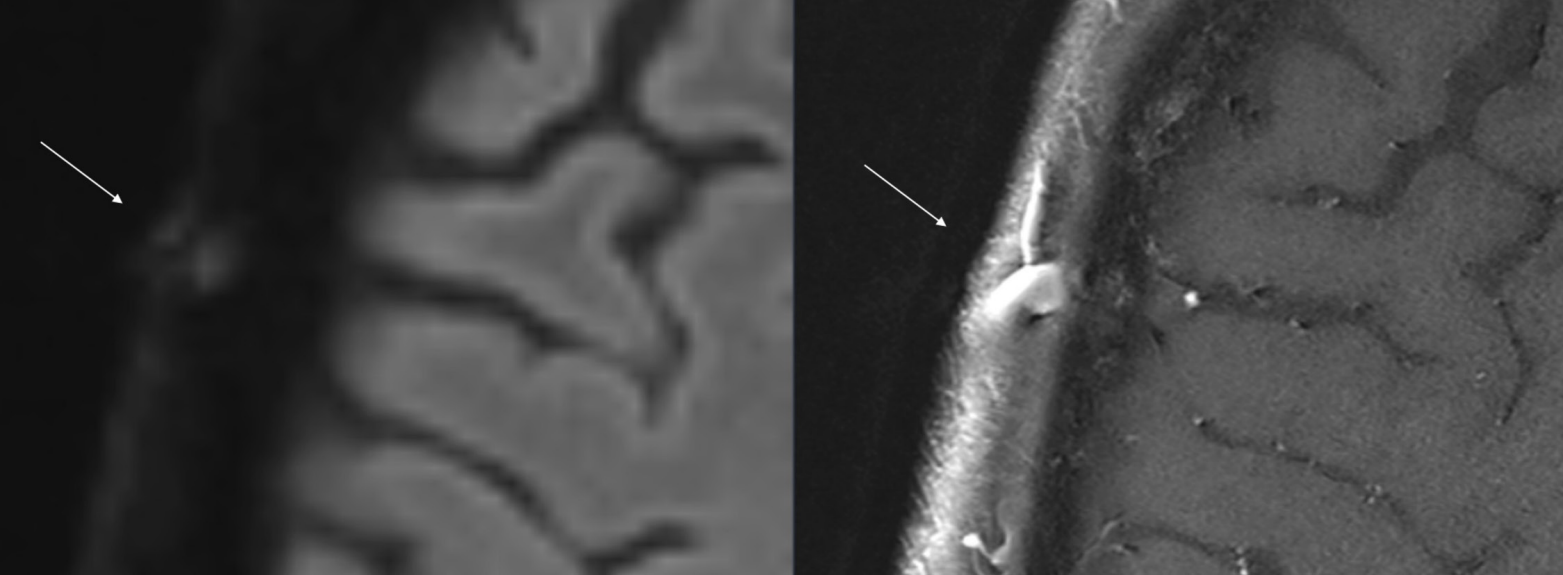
Vein of the scalp with false-positive DSAS: a large vein in the right parietal region shows a DWI-score of 2–3 (left panel). The right panel shows the corresponding T1-black-blood image, where the vein has the typical hyperintense lumen with a large diameter in contrast to the black and smaller lumen of arteries. DSAS, DWI scrolling artery sign; DWI, diffusion-weighted imaging.
